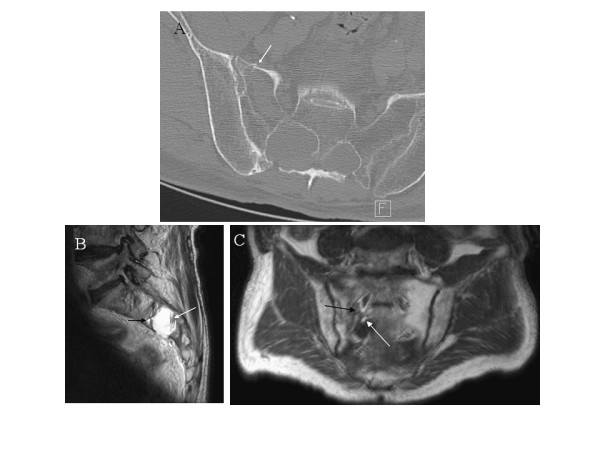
Sacral cyst CT-scan and MRI image. A. Axial sacral CT-scan: left sacral fracture extending to the S2 radicular cyst (presence of a contralateral cyst at the same level). B. Sagittal *T_2_*-weighted sacral MR image: S2 radicular cyst with two liquids: cerebrospinal fluid with blood sediments (white arrow) and fat droplet (black arrow). C. Coronal *T_1_*-weighted sacral MR image: left sacral fracture extending to the radicular cyst (black arrow) which contains cerebrospinal fluid and fat droplets at the bottom (white arrow).
